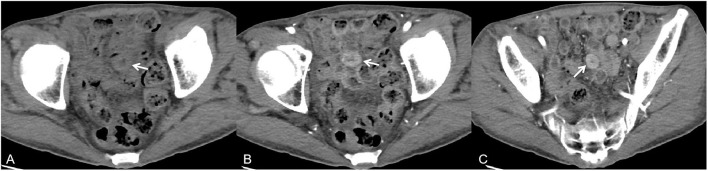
A 57-year-old man presented with cough, sputum, malaise, dizziness or headaches, and abdominal pain for 1 month. Axial non-contrast-enhanced CT imaging showed multiple regions of thickening of the intestinal wall [(A), white arrow]. Axial contrast-enhanced CT images showed lesions with obvious enhancement [(B,C), white arrow].
